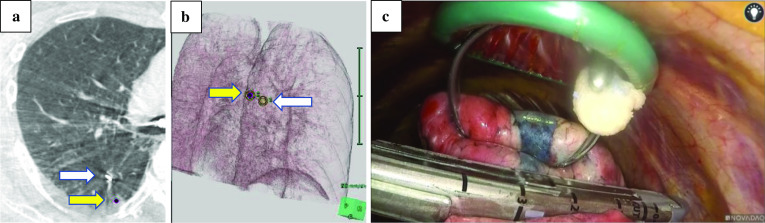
Intraoperative findings of thoracoscopic wedge resection using cone beam computed tomography (CT) in a hybrid surgical theater. a Intraoperative CT image. The yellow arrow indicates the tumor shadow position, and the white arrow denotes the tip of the injection catheter advanced through a working channel of a flexible bronchoscopy. b Three-dimensional positional relationship between the tumor (yellow arrow) and the marking spot on the pleural surface (white arrow), which was reconstructed in a surgical room. c Wedge resection was performed using a linear stapler
